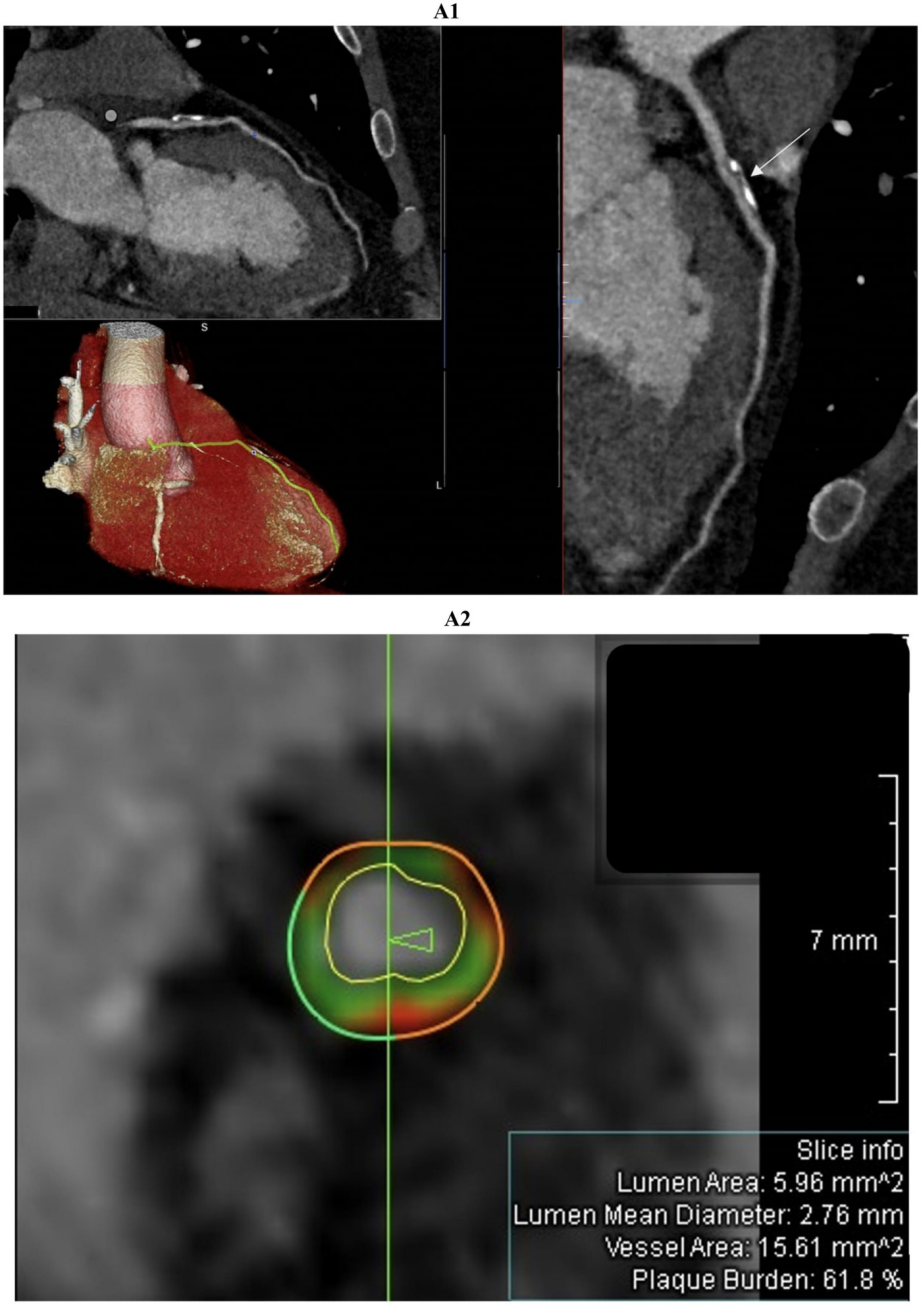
Comprehensive coronary artery evaluation using coronary computed tomography angiography (CCTA) with advanced image reconstructions and quantitative plaque analysis was performed in a 51-year-old male patient admitted with suspected acute myocardial infarction with non-obstructive coronary arteries (MINOCA). The curved multiplanar reconstruction (figure 2A1, top left panel and large right panel) offers a detailed longitudinal view of the left anterior descending (LAD) artery, clearly delineating the vessel lumen and wall morphology. The proximal LAD segment reveals an extensive, eccentrically located plaque predominantly composed of calcific tissue. This lesion exhibits typical features of positive remodeling without causing significant luminal narrowing or flow limitation. The three-dimensional volume-rendered image (figure 2A1, bottom left panel) shows the heart and coronary arteries with the LAD artery highlighted in yellow, facilitating spatial comprehension of plaque localization and vessel trajectory from the left main coronary artery to the apex. Cross-sectional imaging (figure 2A2), indicated by the white arrow in figure 2A1, combined with quantitative coronary plaque analysis identifies a high-risk plaque with positive remodeling. The green vertical line marks the exact location of the cross-section shown in figure 2A2 and the arrow indicates the slice orientation relative to the vessel. This concentric plaque includes dark green fibrous tissue, light green fibrofatty tissue, and red necrotic core, which are characteristic of high-risk features. The external vessel wall contour is partly dark green, representing fibrous tissue, and partly red, indicating the necrotic core. The internal vessel wall contour, shown in light green, corresponds to fibrofatty tissue. The white boundary inside the vessel marks the lumen contour, defining the blood flow space. Lumen area (5.96 mm^2^), mean diameter (2.76 mm), vessel area (15.61 mm^2^), and plaque burden (61.8%) are reported in the slice info box.
